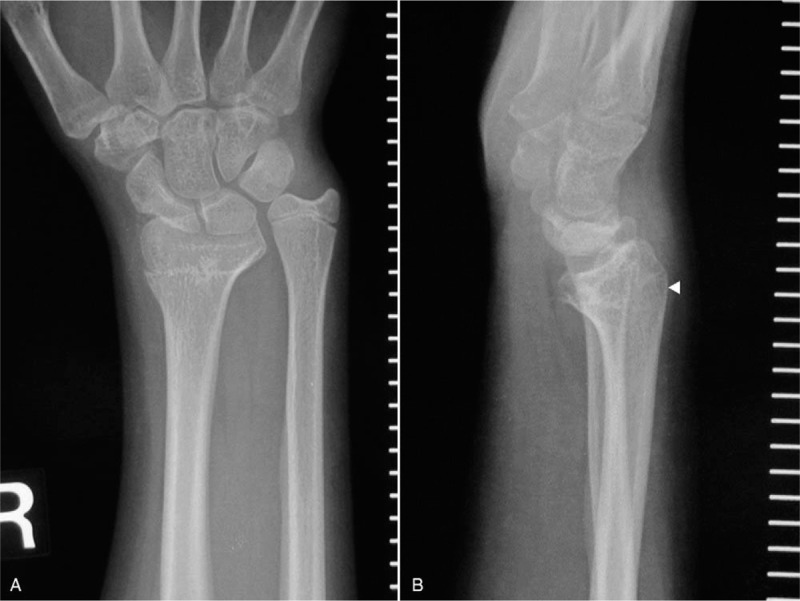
Radiographs taken at the time of presentation showing the right forearm of a 14-year-old girl with painful functional limitation due to radioulnar discrepancy and dorsal subluxation of the ulna. (A) Anteroposterior view. (B) Lateral view.
